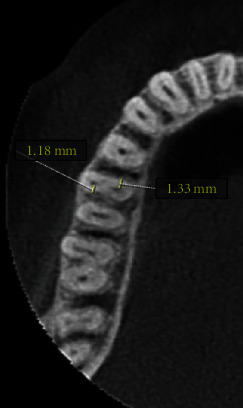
Measurement on the distal wall of the mesiobuccal and the mesial wall of the mesiolingual canals in a right mandibular first molar on axial CBCT image.
